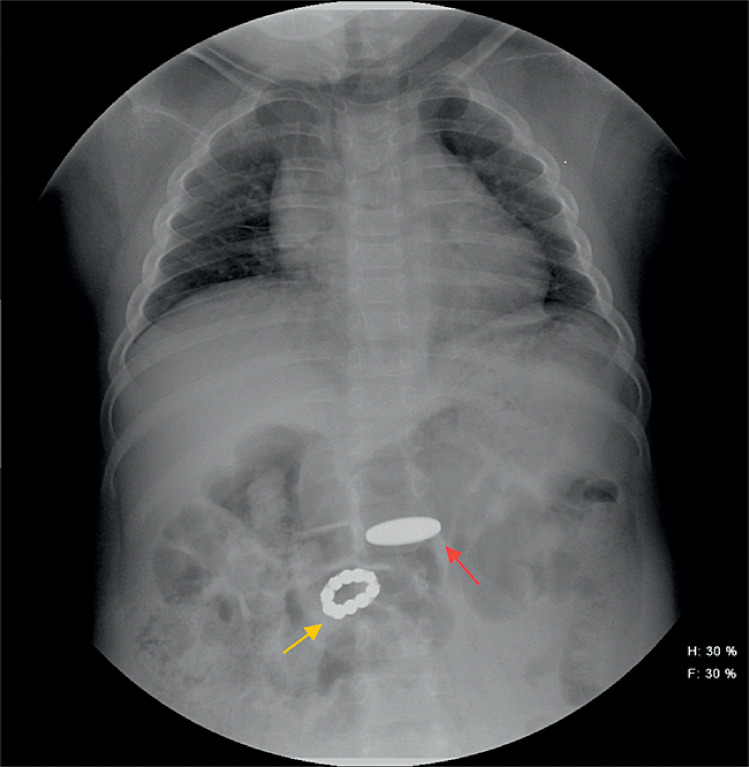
Abdominal X-ray showed the image of the coin in the stomach (red arrow), and a string of beaded, high-density images, forming a ring, in the right upper abdomen (yellow arrow).
